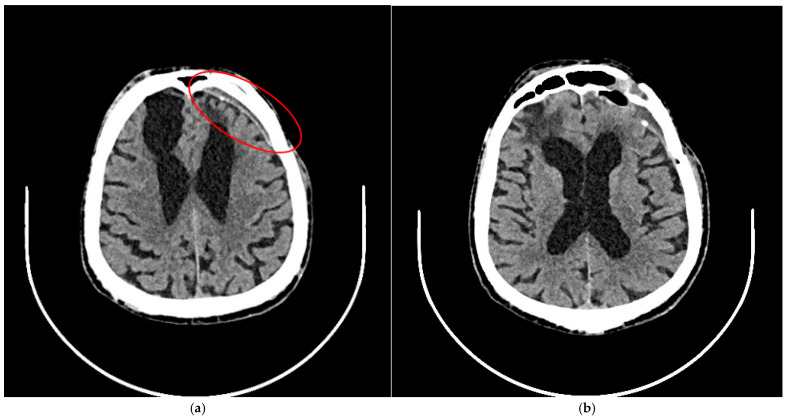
A follow-up CT scan six months after treatment showed no evidence of new intracranial hemorrhage. (a) A narrow chronic subdural hematoma was noted (red circle). (b) Extensive hypodense post-traumatic changes were observed at the base of both frontal lobes. The ventricular system was significantly enlarged and asymmetrical, with evidence of right-sided retraction. There was no displacement of midline structures.
